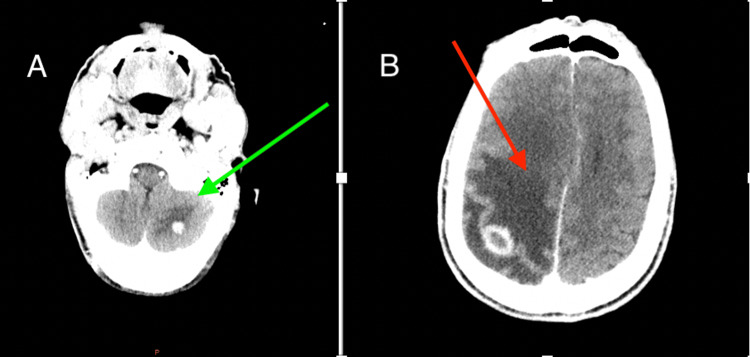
CT brain scan A: cortico-subcortical hypodense area indicates perilesional edema with a mass effect on the ipsilateral ventricle and a 9-mm midline shift to the left (green arrow); B: intra-axial lesions in the supra- and subtentorial regions; the largest lesion is located in the right parietal region, suggesting secondary lesions (red arrow).
